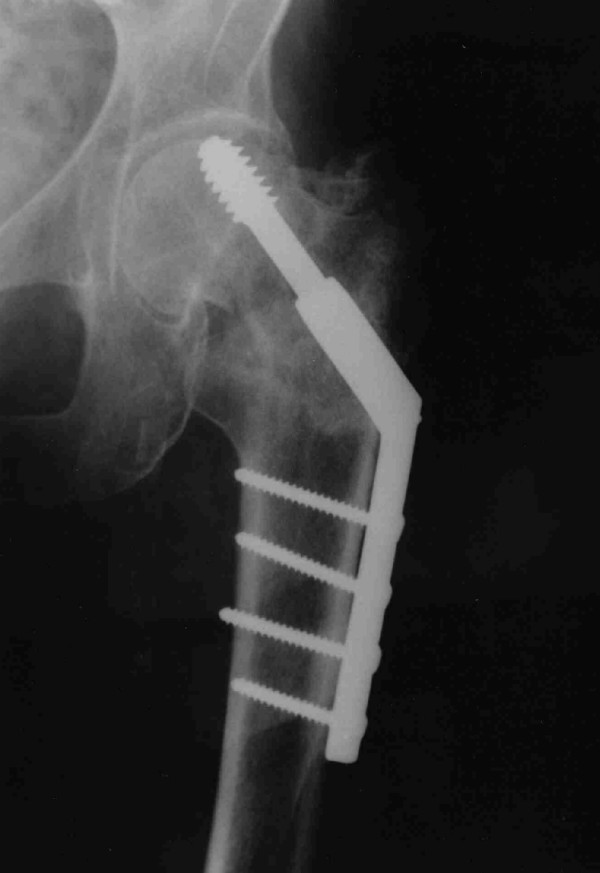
AP Radiograph of left hip depicting failure of fixation and evidence of an intracapsular fracture.
